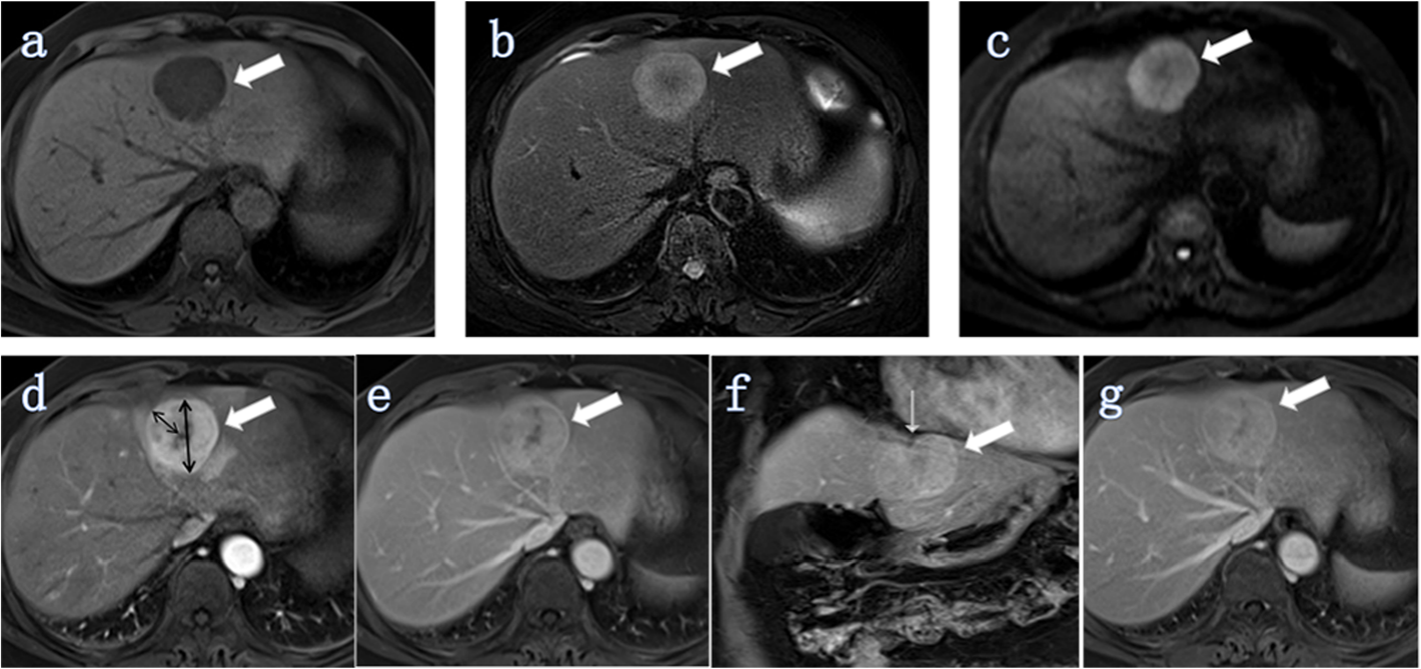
ICC (white thick arrow) without MVI in a 65-year-old woman (tumor grade, G2; CA19–9, ≥37 U/ml). Intrahepatic duct dilatation and visible hepatic artery penetration did not appear in all images of this lesion. a Axial T1-weighted image showing a tumor located in segment IV of the liver. The tumor morphology was spherical. b Axial T2-weighted-FS image showing that the signal of tumor edge was high intensity while that of the tumor center was low intensity. c Axial DW image (b = 500 s/mm^2^) showing a higher signal with a target sign. d Axial arterial phase image showing that the tumor exhibited edged enhancement (ring high signal). The maximum diameter of the tumor was 4.69 cm and the maximum enhancement edge thickness was 1.90 cm (black double arrows); the arterial edge enhancement ratio was 40.51% (1.90/4.69). e Axial portal vein phase showing the range of strengthening increased and began to fill the center of the lesion. f Coronal portal venous phase showing retraction of the hepatic capsule adjacent to the tumor (white thin arrow). g Axial delayed phase image showing that the signal of the tumor was high and the enhancement ratio of the lesion was approximately 4/4 (3/4 ≤ R ≤ 4/4)
